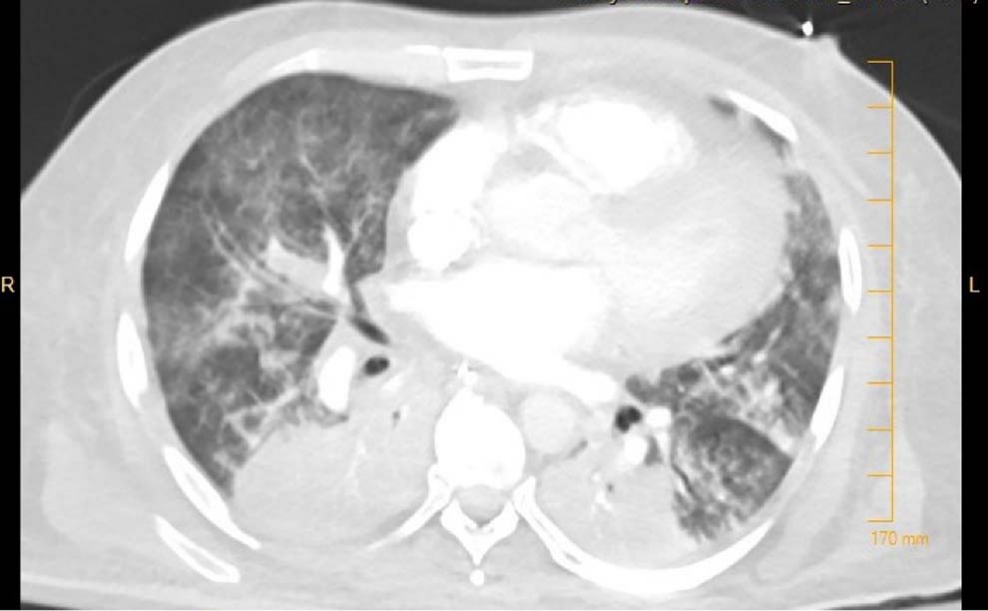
Computed tomography angiogram shows extensive bilateral pulmonary consolidation as a result of ventilator-associated pneumonia coinfection of *Burkholderia gladioli* and coronavirus disease 2019 (COVID-19).
